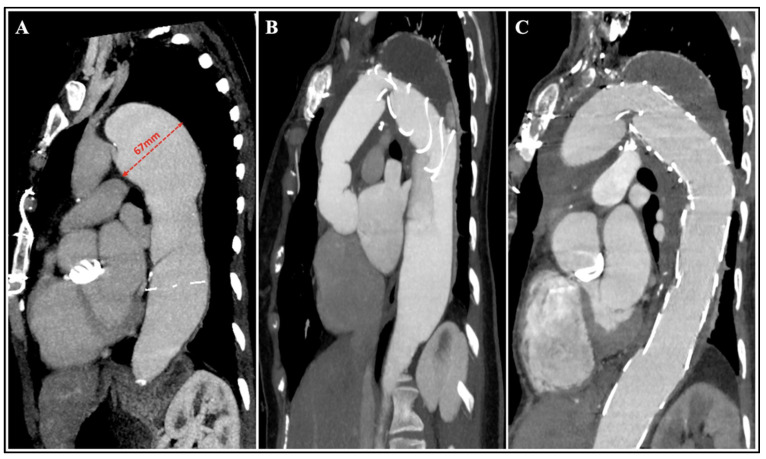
Multi-stage approach. CTA follow-up of a 68-year-old male patient with a complex aneurysm of the descending aorta involving the aortic arch, which developed 15 years after a previous mechanical Bentall procedure for aortic root aneurysm (A). The patient underwent redo TAR-FET as a first-step procedure (B), followed by second-stage TEVAR completion 4 months thereafter (C), allowing total exclusion and thrombosis of the aneurysmal sac without endoleak. CTA: computed tomography angiography; FET: frozen elephant trunk; TAR: total arch replacement; TEVAR: thoracic endovascular aortic repair.
